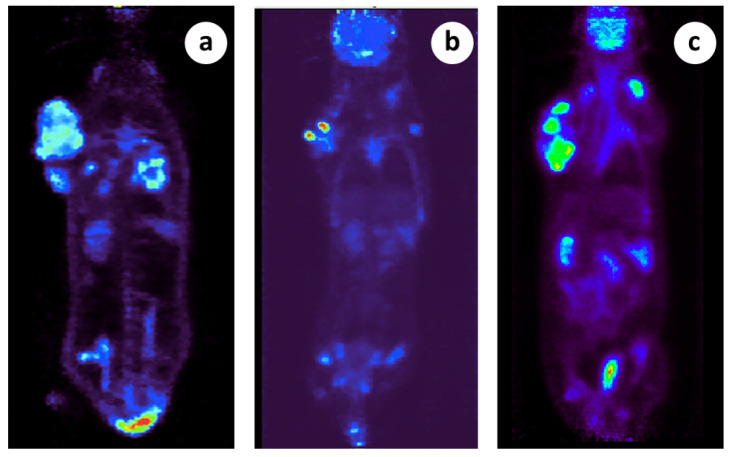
Micro PET scanning demonstration of primary and metastatic lesions in 3 different subjects. (a) There is a large tumor located in the right thoracic wall posteriorly demonstrating heterogeneous tracer distribution (indicative SUVmax: 3.9) and photopenic areas indicative of necrosis. Also, there is a second soft tissue mass in the right lateral chest wall close to the aforementioned large tumor likely representing a regional lymph node (SUVmax 4.2). In addition, there are multiple metastatic lung lesions bilaterally (indicative SUVmax 3.9), (b) There is a large tumor located in the right thoracic wall posteriorly demonstrating heterogeneous tracer distribution (indicative SUVmax: 11.2) and photopenic areas indicative of necrosis. In addition, there is a second soft tissue mass in the right lateral chest wall close to the aforementioned large tumor likely representing a regional lymph node (SUVmax 9.7). Focal FDG uptake (SUVmax 2.4) is also noted in what appears to be an additional lymph node in the left side of the thoracic cage superiorly, (c) There is a large tumor located in the right thoracic wall posteriorly demonstrating heterogeneous tracer distribution (indicative SUVmax: 8.8) and photopenic areas indicative of necrosis. Also, there is a second soft tissue mass in the right anterolateral chest wall close to the aforementioned large tumor likely representing a regional lymph node (SUVmax 10.0).
